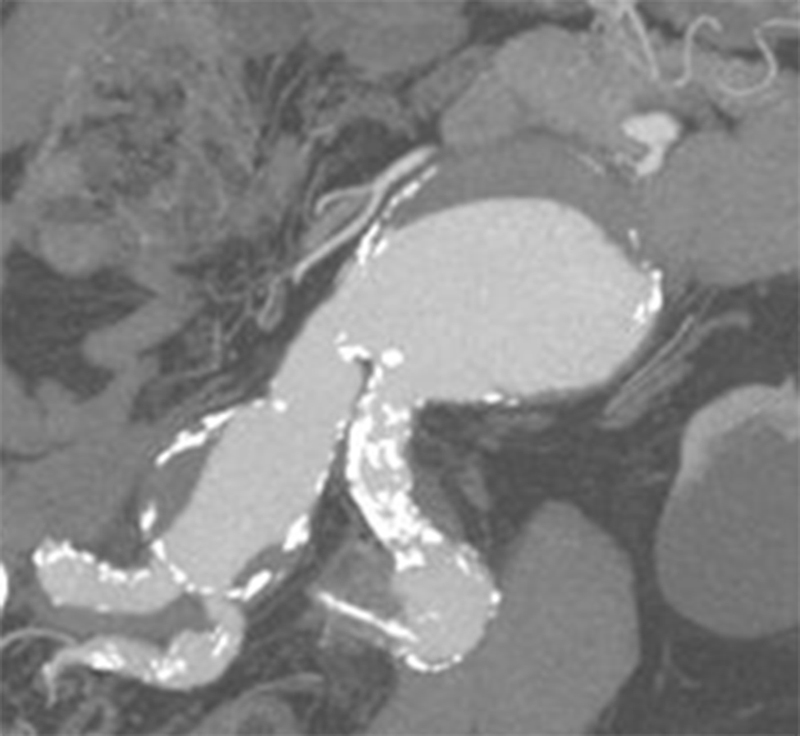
Computed tomography angiography showing an abdominal aortic aneurysm of 8 cm in diameter extending in both iliac arteries.
